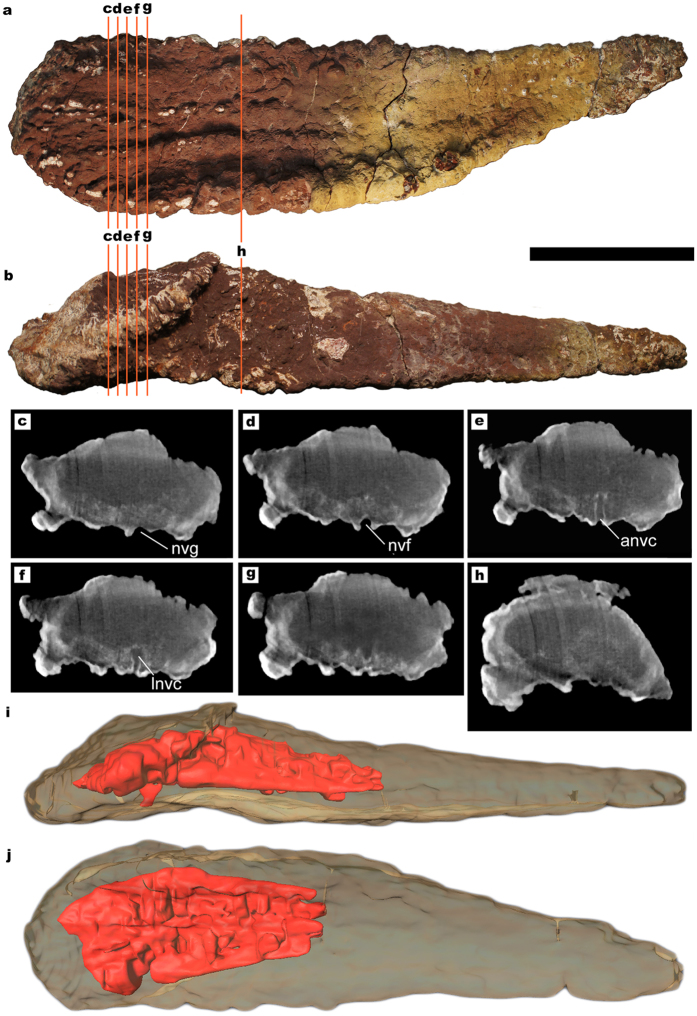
Titanosaur osteoderm with large internal cavities. (a) HUE-02000 in deep view. (b) HUE-02000 in lateral view. (c–h) CT-scan slices at different positions, where the lower density area and the neuro-vascular canal can be observed. Notice how the neurovascular canal walls are well differentiated by the iron mineral crust covering it, and that is denser than any material in the interior of the osteoderm. (i) 3D model of HUE-02000 in lateral view, obtained from the CT-scan slices, with the void and canal in red. (j) 3D model of HUE-02000 in deep view. Scale = 100 mm. anvc = ascending neurovascular canal. lnvc = longitudinal neurovascular canal. nvf = neurovascular foramen. nvg: neurovascular groove. ol = ossified ligament.
